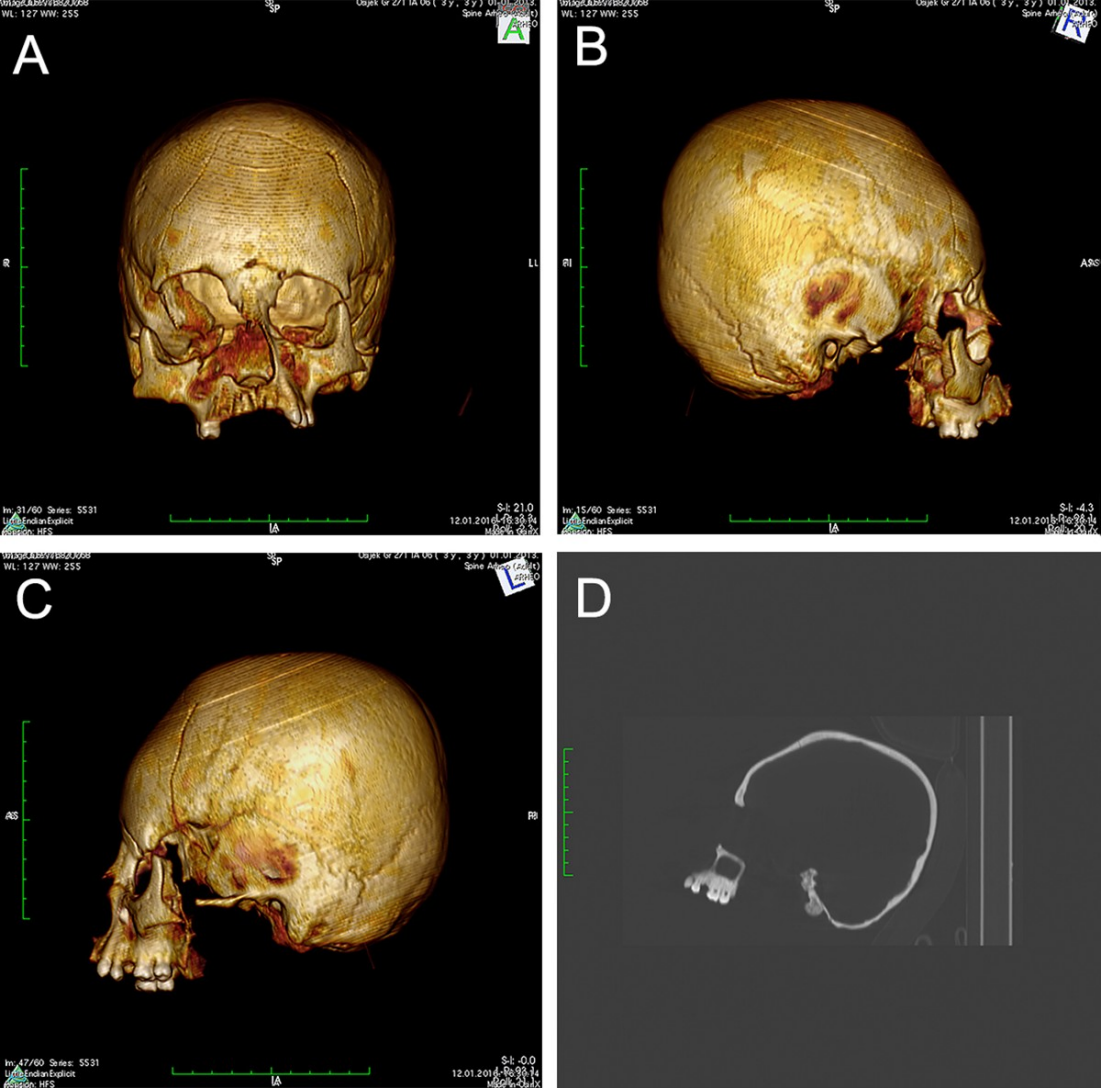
A-C) CT reconstruction showing the artificially deformed cranium belonging to individual SU 751, frontal and lateral views. A pronounced flattening of the frontal bone resulting in a remarkable growth of cranium height suggests the circular erect type of ACD. D) The flattening and significant thickening of the frontal bone anterior of bregma is visible on the X-ray image (lateral view).
